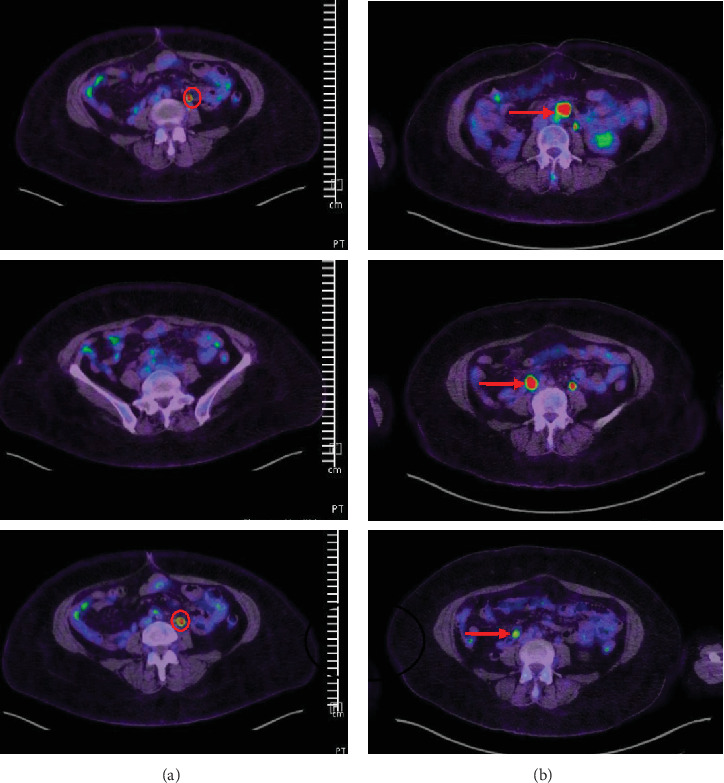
FDG PET scans in January 2025 (a) compared to October 2019 and April 2020 (b): The red arrows indicate metabolic activity in the right common iliac lymph nodes and retroperitoneal lymph nodes. The red circle denotes physiological uptake and excretion in the left ureter. The FDG PET scan from January 2025 (a) demonstrates sustained resolution of the retroperitoneal and right common iliac lymph nodes.
